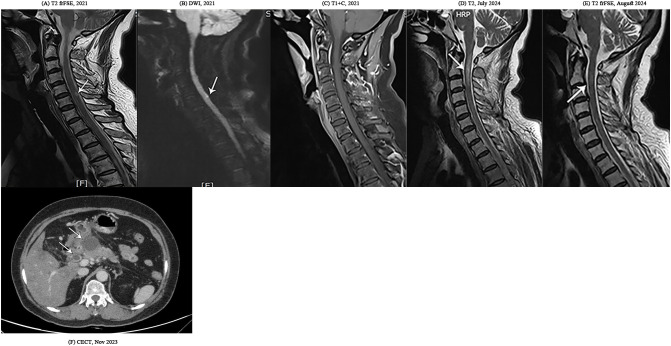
The 2021 MRI showed a nodular intramedullary hyperintensity at the C5–C6 level on T2-weighted (A) and DWI images (B), without significant enhancement on the contrast-enhanced image (C). (D) The July 2024 MRI revealed a new longsegment T2 hyperintensity within the spinal cord at the C1–C3 levels, concurrent with the resolution of the previously observed space-occupying lesion. (E) Follow-up MRI in August 2024 demonstrated marked regression of the C1–C3 long-segment lesion. (F) Contrast-enhanced CT on November 27, 2023, revealed multifocal lesions in the pancreatic head, body, and tail, including a cystic lesion in the pancreatic neck, all suspicious for neoplasm. Additionally, diffuse abnormal contrast enhancement was observed in the pancreatic segment of the common bile duct wall, also suggestive of neoplasm and resulting in biliary obstruction.
